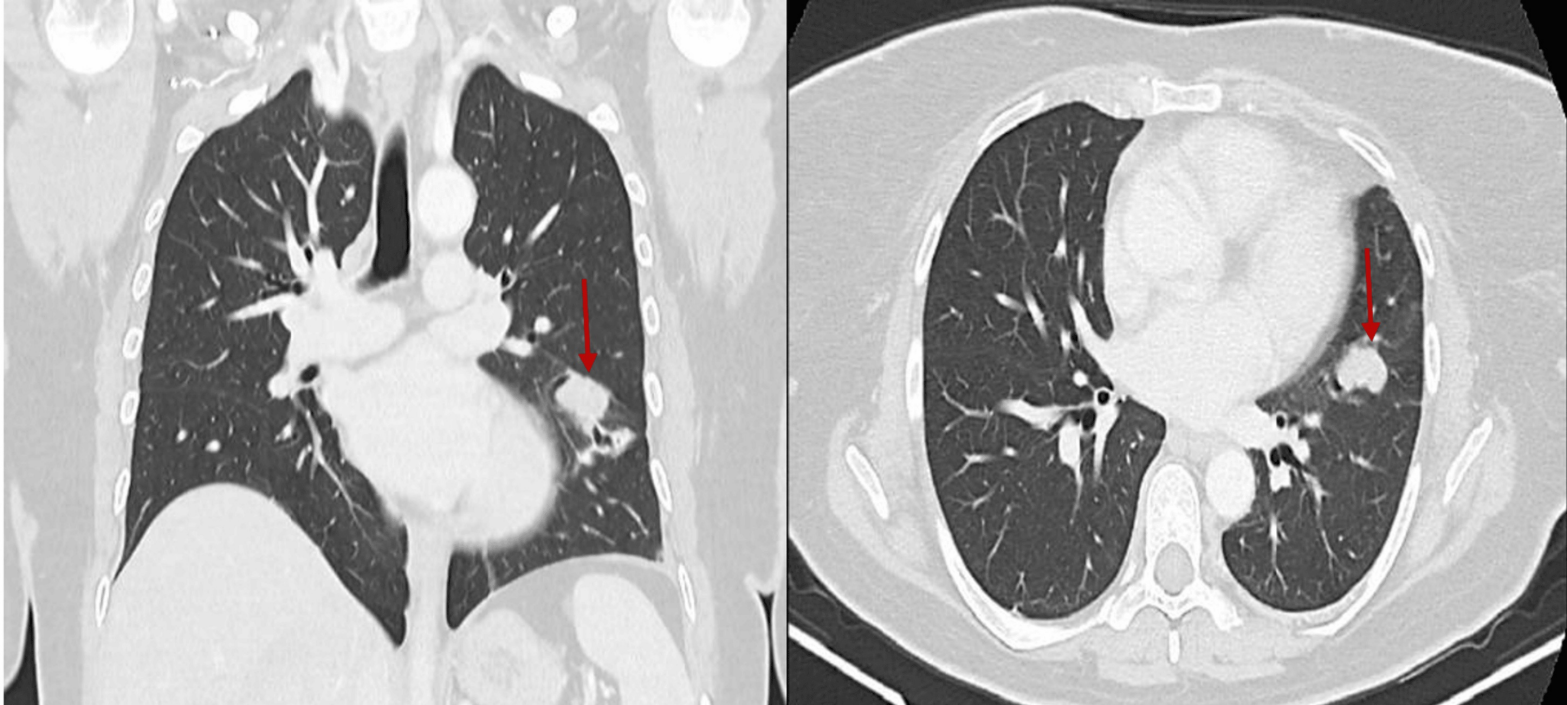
Thoracic CT scan revealed a 25x15 mm, well-defined nodule in the lingula
